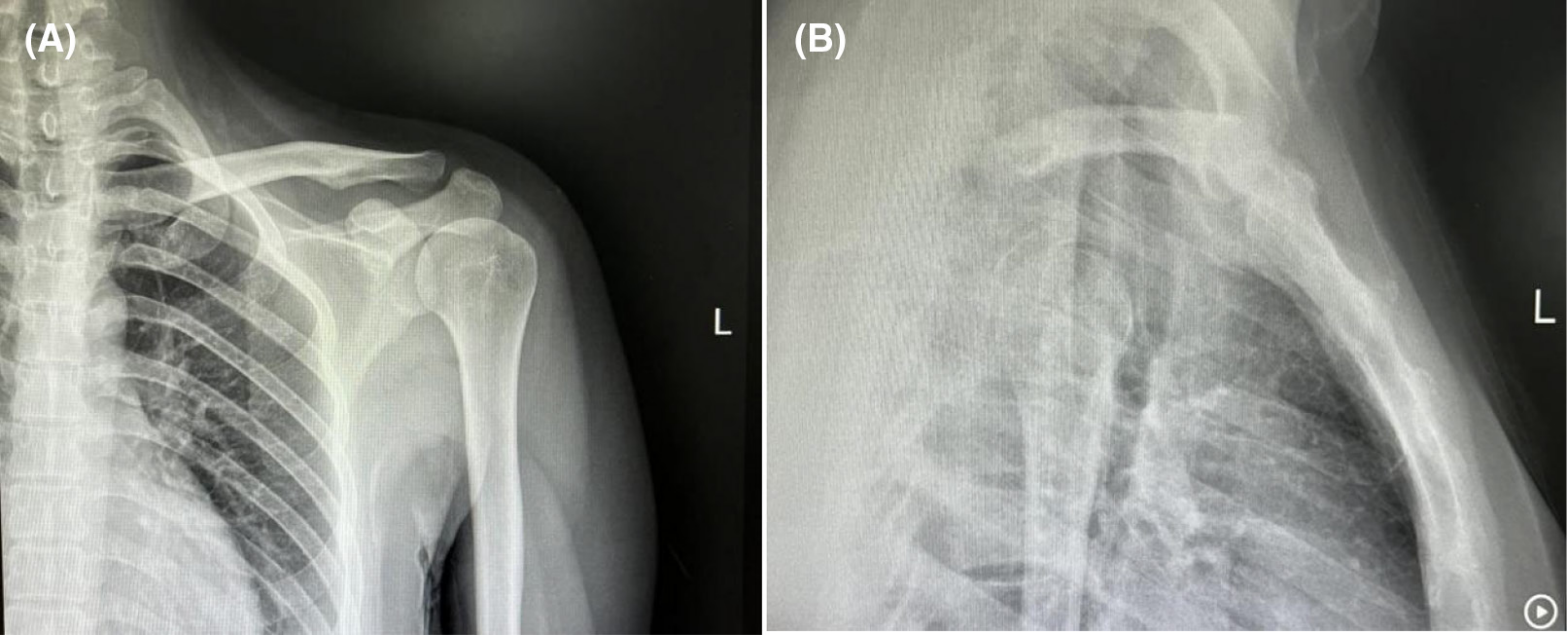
(A) & (B) Radiographs immediately after surgery showed that the osteochondroma was completely resected.
